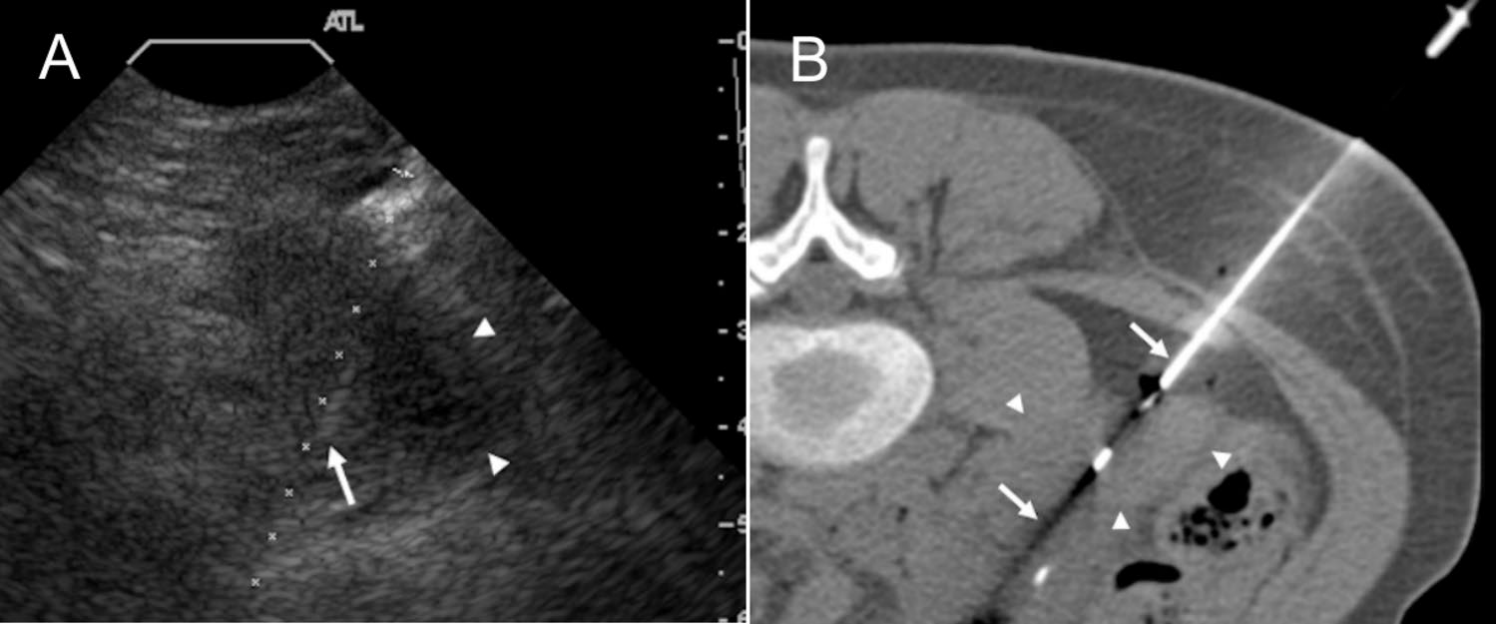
Image-guided biopsy for rejection. The Ultrasound (A) and computed tomography image (B) shows a longitudinal biopsy trajectory (arrow) along the long axis of the pancreas graft (arrowhead) to avoid vascular and ductal injury. Biopsy confirmed mild acute rejection
